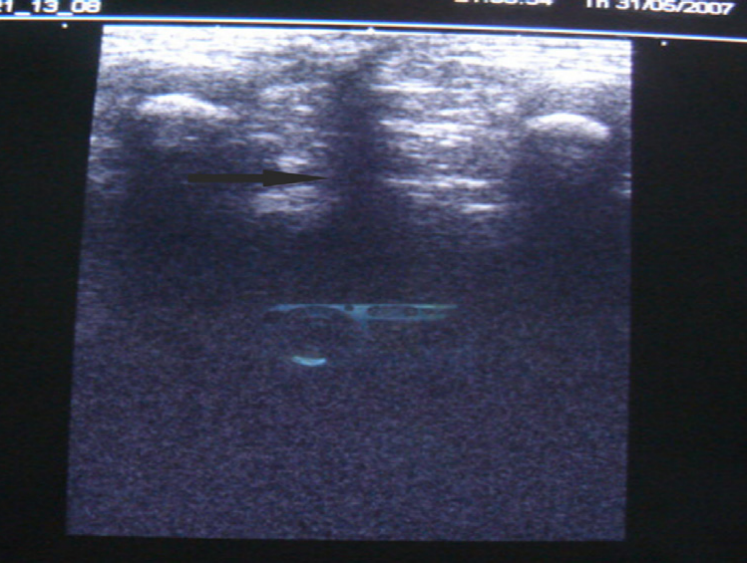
Sonogram showing guide wire (arrow) in the center of the muscle complex.
